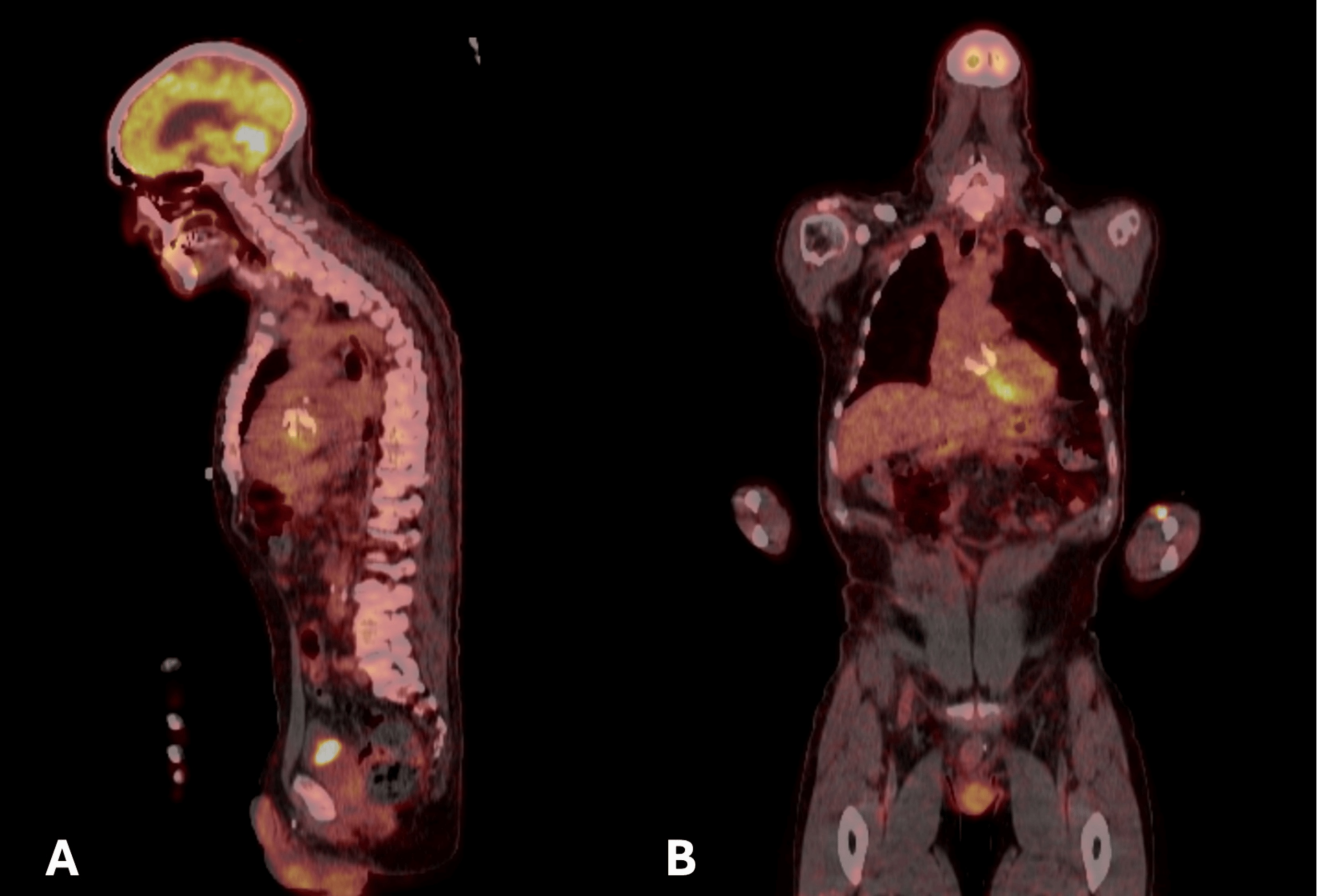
18F-FDG positron emission tomography/computed tomography images showing no evidence of infective endocarditis: (A) sagittal plane; (B) coronal plane. There was no tracer accumulation at the aortic valve replacement to suggest an underlying infective process. Left ventricular myocardial uptake was reported as physiological.
